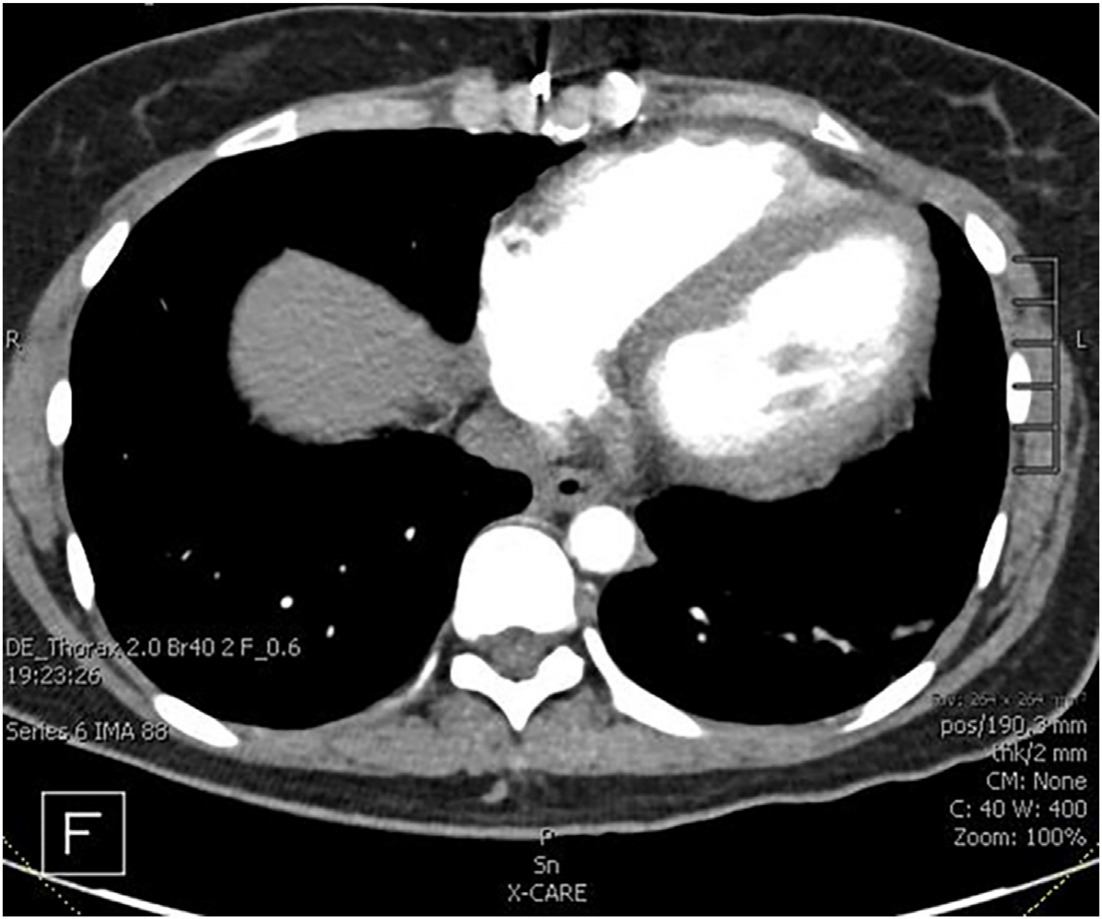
Preoperative computed tomography scan showing broad surface of contact of right and left ventricle to left anterior chest wall.
